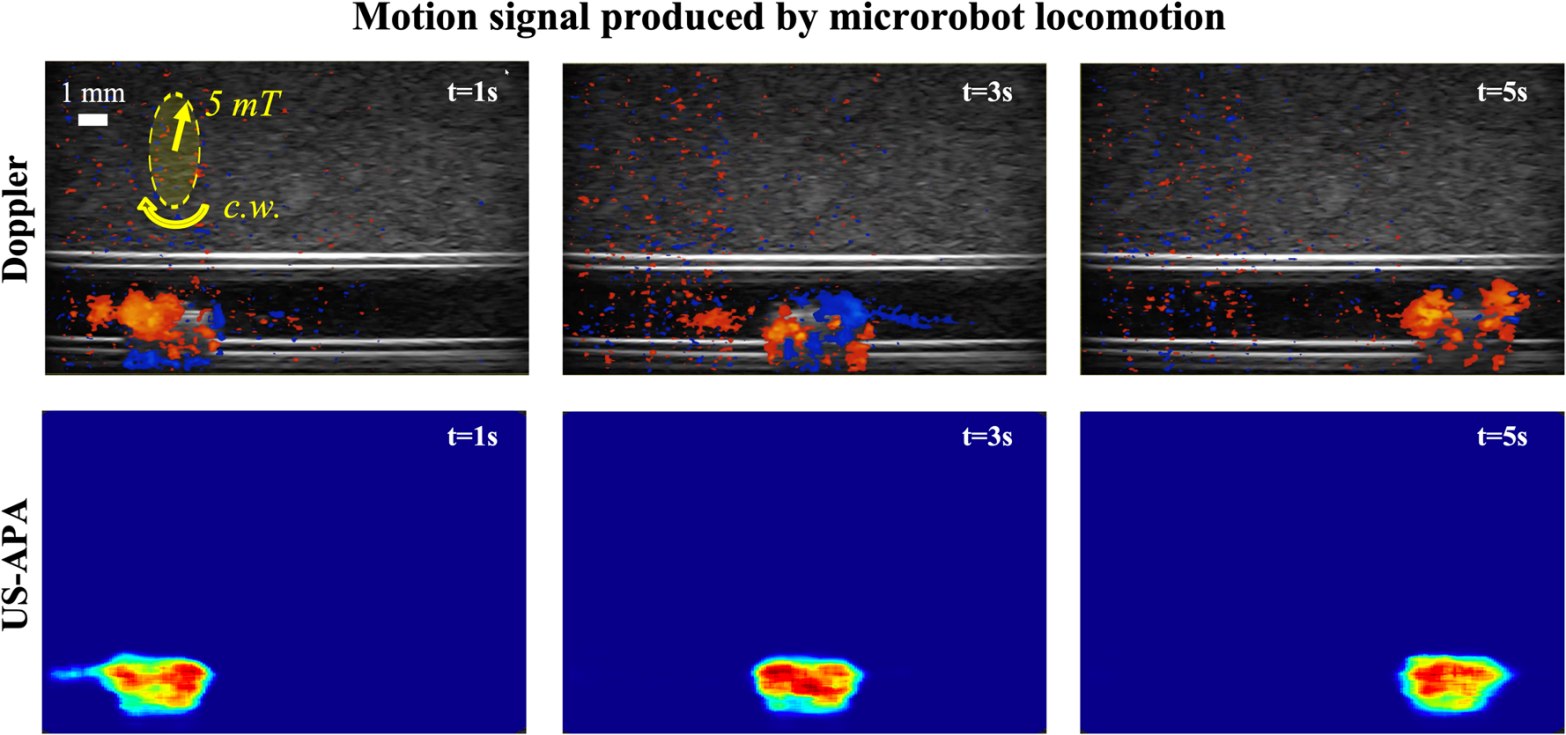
Imaging experiments during locomotion in a straight lumen. Yellow arrows represent the field intensity and rotation direction (c.w. stands for clockwise). The motion signals obtained with color Doppler and US-APA images of the same region are compared at different time points along the MR trajectory. The specific helical propeller motions successfully enhance its contrast in US-APA images with respect to other high echogenic elements (e.g., white lumen boundaries in Doppler images).
